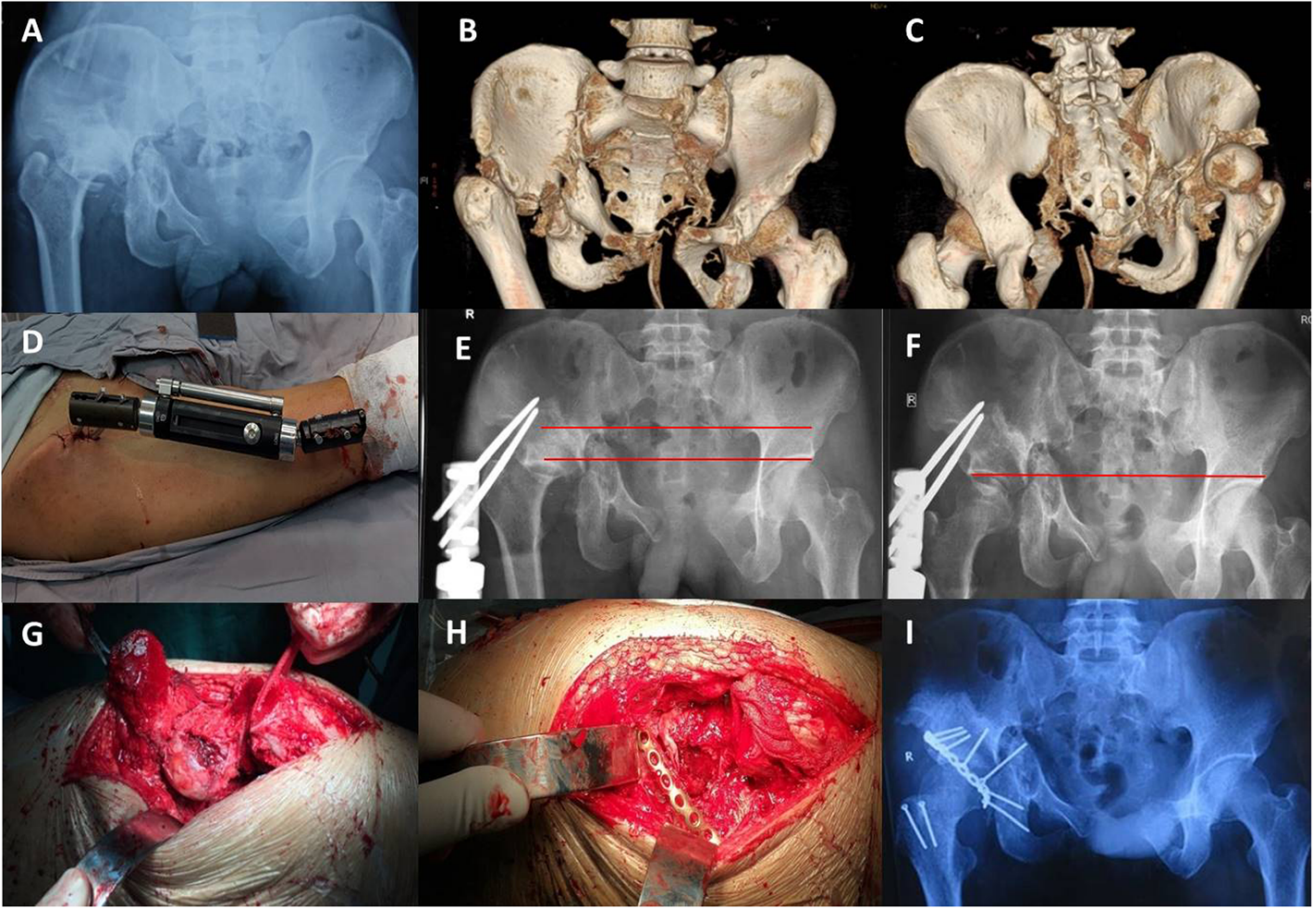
Patient 12. a-c Preoperative X-ray and three-dimensional reconstruction computed tomography images 6 months after injury. d-e External fixation placement. f The femoral head was drawn underneath the articular surface after 31 days of traction. g-h, k-l incision, open reduction with osteotomy of the greater trochanter, and fixation of posterior wall of acetabulum. i Postoperative X-ray image showed correction of limb length discrepancy and reduction of femoral head was originated from our previous study [16] and was authorized by Chinese Journal of Orthopaedic Trauma
